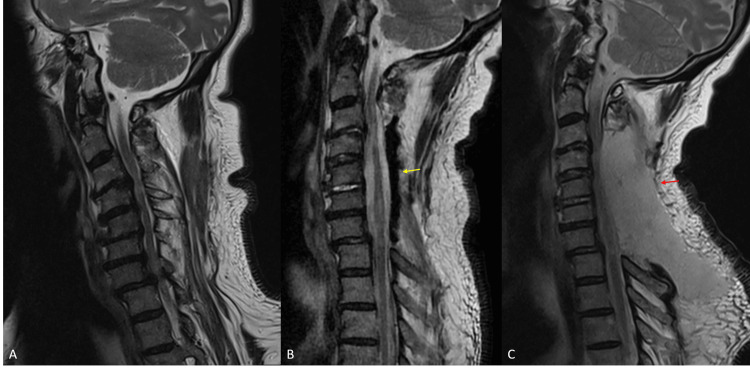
(A) Preoperative MRI demonstrating multilevel cord compression with mild kyphosis. (B) Immediate postoperative MRI demonstrating adequate posterior decompression (yellow arrow) and improved alignment. (C) MRI on postoperative day 11 showing a large compressive seroma (red arrow).
